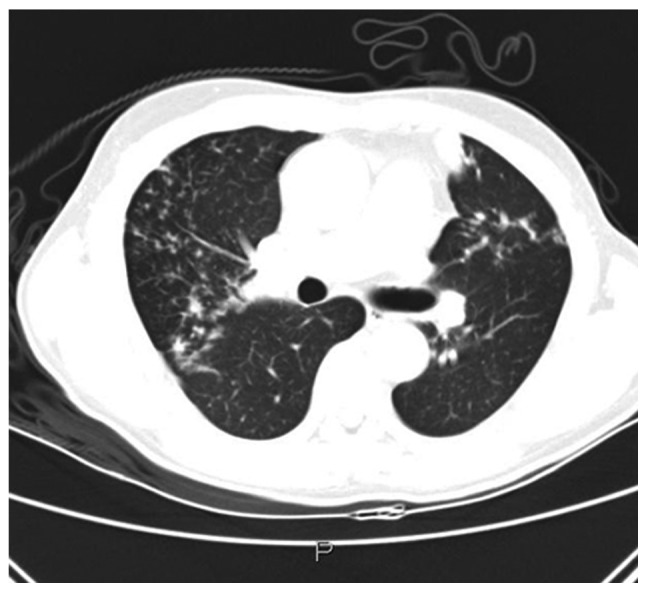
Contrast-enhanced computed tomography (CT) chest scan at two months after the initiation of anti-tuberculosis therapy (April, 2011) demonstarting a decrease in the number of nodules and intrapulmonary patches of varying sizes.
